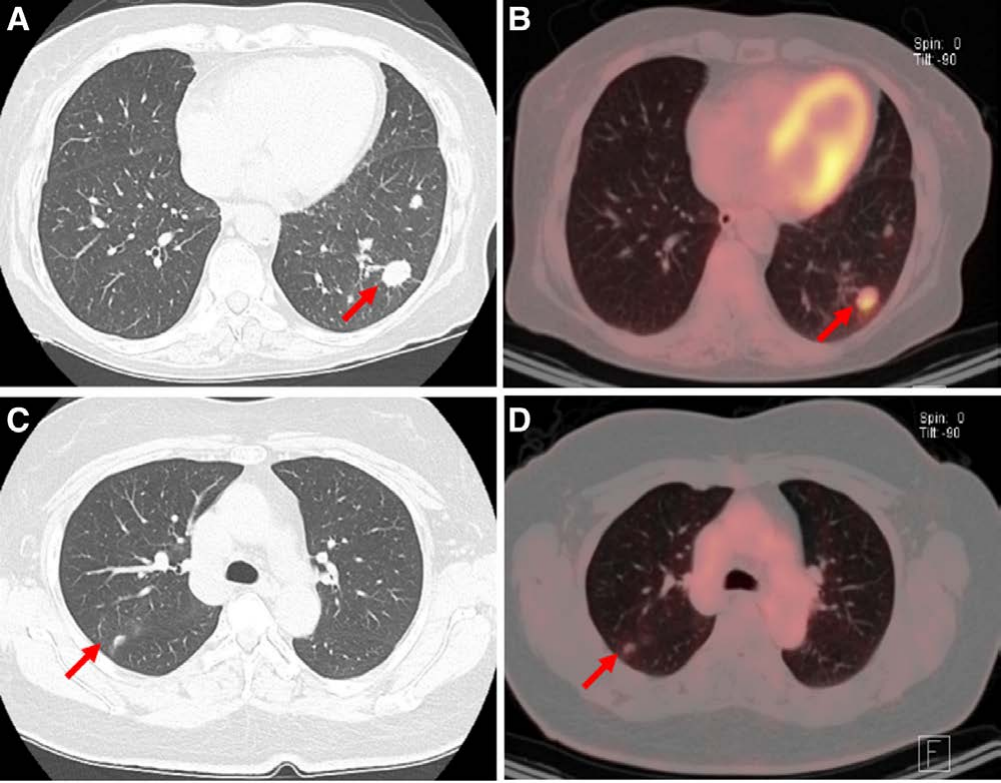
Radiological findings. Computed tomography revealing multiple nodules with lobulated margins and mild enhancement in the left lower lobe (A) and several small nodules in the right upper lobe (C). Positron emission tomography-computed tomography images demonstrating increased FDG uptake in the nodules (B and D). FDG = fluorodeoxyglucose.
